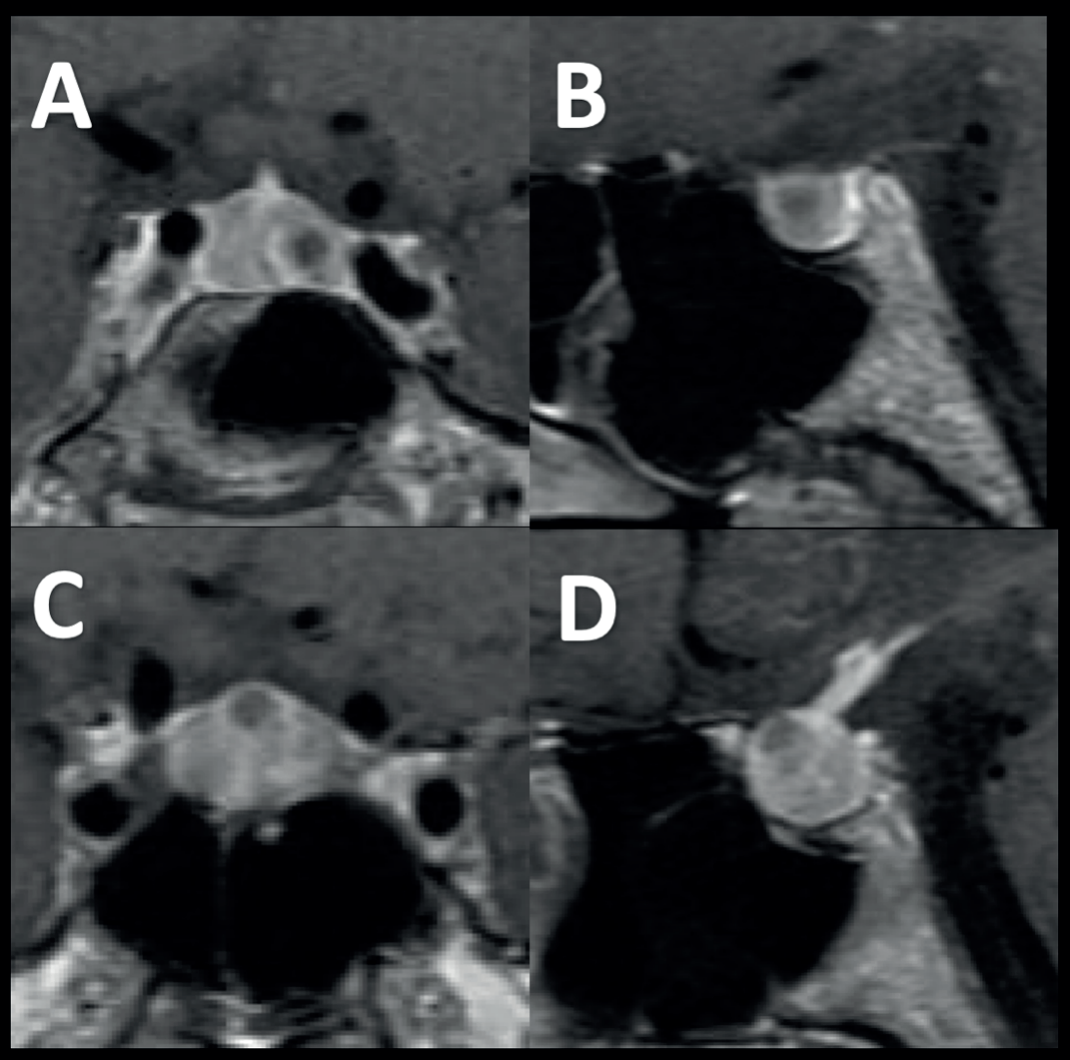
Magnetic resonance imaging (MRI) scan. Coronal and sagittal T-1 weighted MRIs showing an inferolateral pituitary tumor (images A and B) separated from a superomedial pituitary tumor (images C and D).
